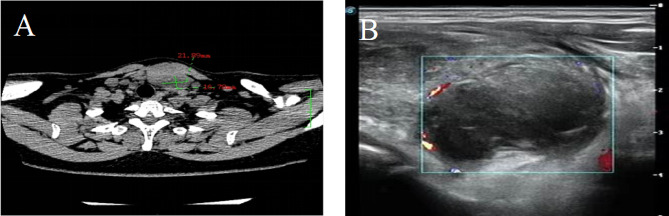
A - Computerized tomography (CT) scan showing a low-density mass measuring 2.1 cm × 1.7 cm in the left thyroid lobe. B - Thyroid ultrasound revealing a solid hypoechoic nodule measuring approximately 2.0 cm × 1.8 cm with unclear borders, irregular shape, and non-uniform internal echogenicity in the left thyroid lobe
